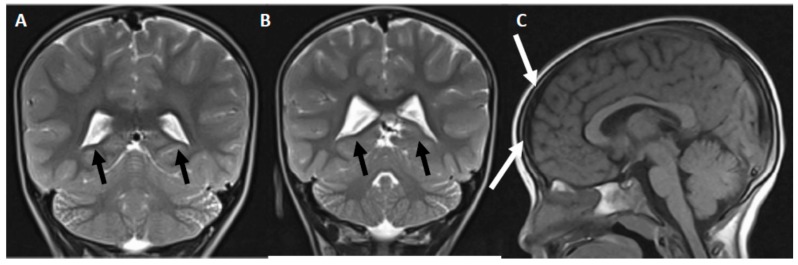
Brain structural alterations observed in the patient. (A,B) Axial, magnetic resonance, T2-weighted images showing discrete asymmetry of the lateral ventricles. Note that the right side is larger (indicated by the black arrows). (C) Sagittal, magnetic resonance, T1-weighted image showing discrete frontal subdural hygroma (indicated with white arrows).
